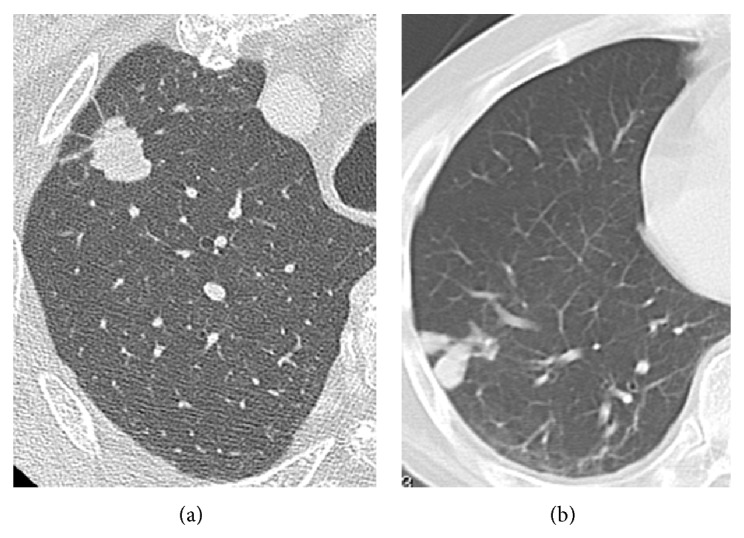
Representative chest CT images of pulmonary cryptococcosis of the nodule or mass without GGA/consolidation type. One patient showed a solid nodule with spiculation in the right upper lung (a). Another patient showed plural nodules, which had contact with the pleura in the right lower lobe (b).
